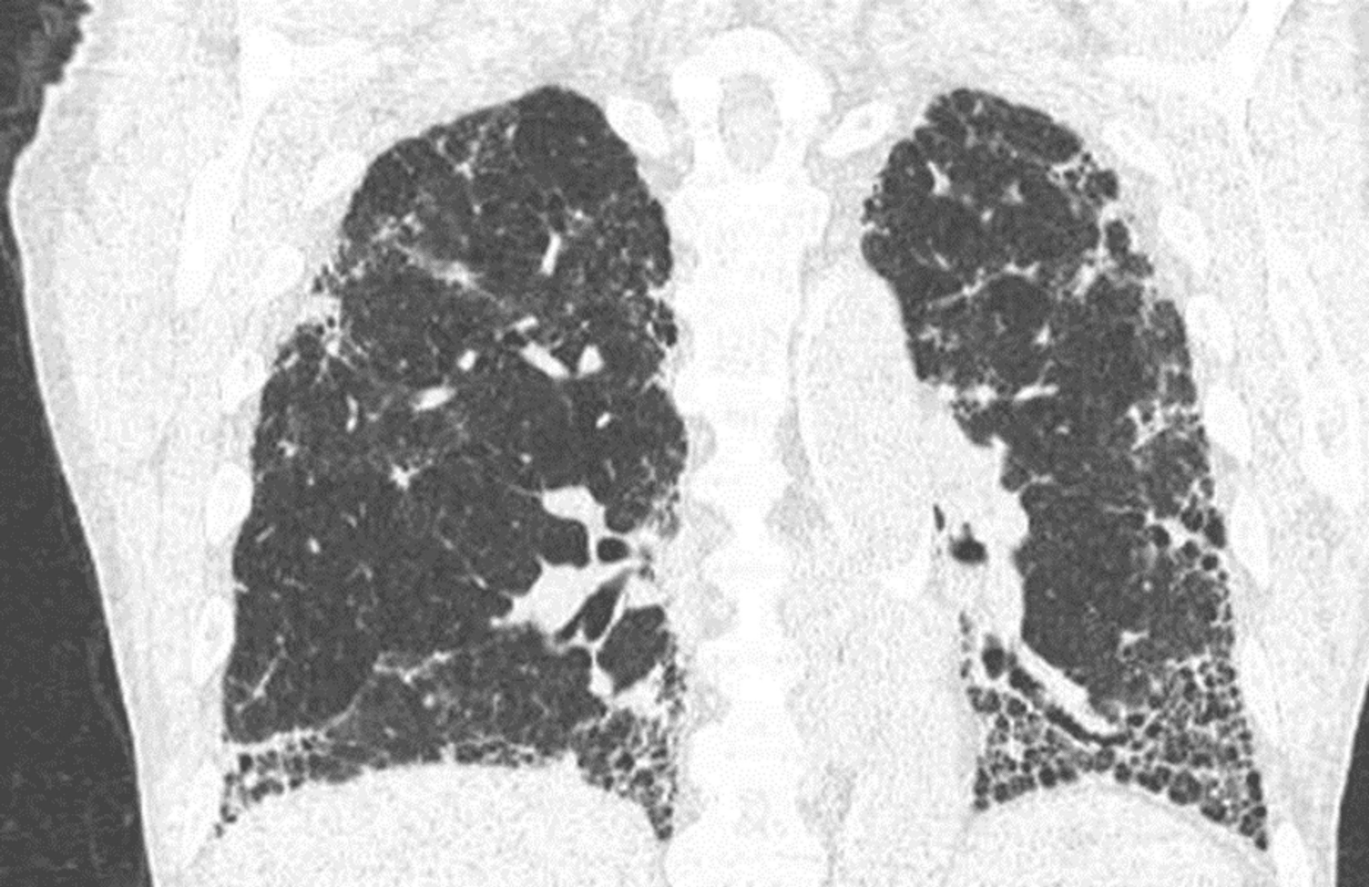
Coronal CT chest with no contrast highlighting lower lobe-predominant UIP features. Coronal high-resolution CT image of the chest showing a fibrotic change of usual interstitial pneumonitis pattern; peripheral reticulation, interseptal thickening, traction bronchiectasis, traction bronchiolectasis, architectural distortion, honeycombing, and volume loss. UIP: usual interstitial pneumonia
